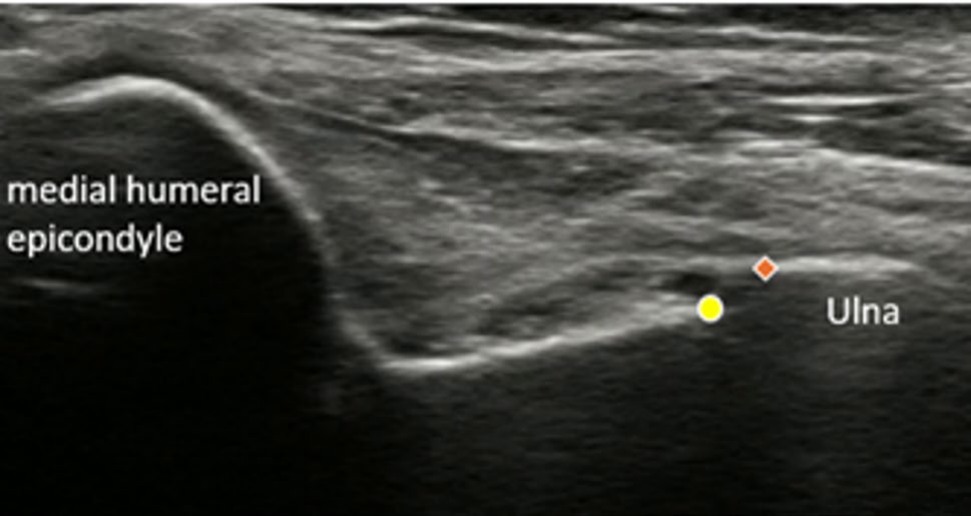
Representative ultrasound image showing medial elbow joint space measurement. The humeral side of the joint space is marked with circles (○), and the ulnar side is marked with diamonds (◇)
